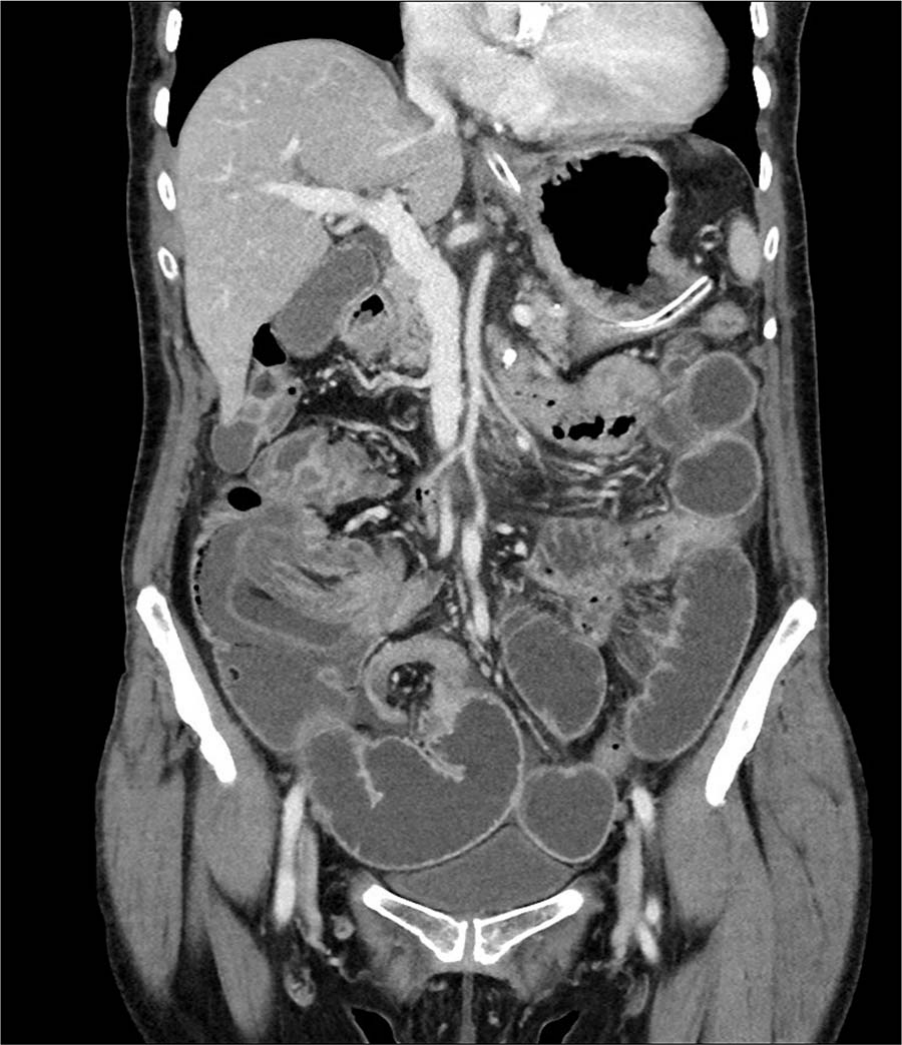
Coronal abdominal CT scan.
